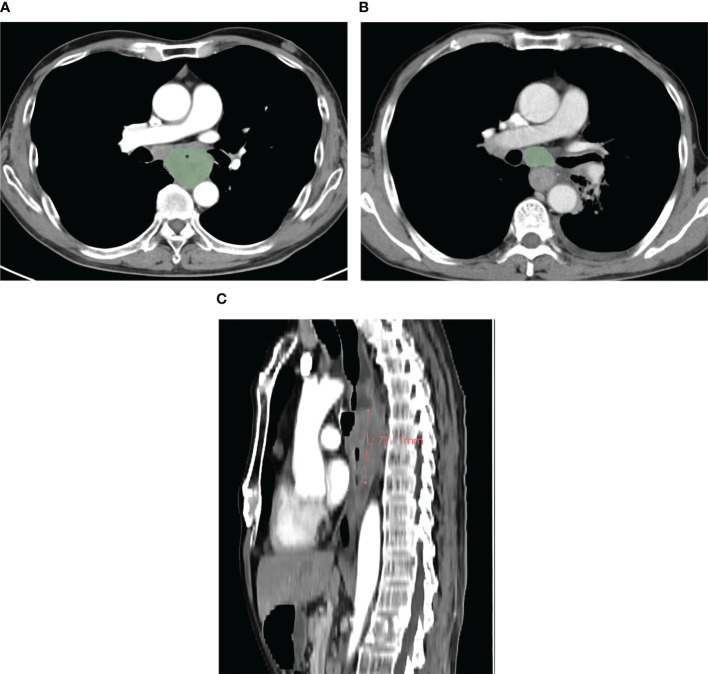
In a 59-year-old male patient with cT_4_N_1_M_0_ esophageal squamous cell carcinoma, the pretherapeutic contrast-enhanced CT scans show the gross tumor volume obtained by slice-by-slice manual delineation of the tumor (A), and the gross tumor volume is 57.66 cm^3^. In a 64-year-old male patient with esophageal squamous cell carcinoma at cT_3_N_1_M_0_, the gross volume of the enlarged lymph nodes was obtained in a way similar to that of the tumor (B), and the gross volume of all enlarged lymph nodes is 21.28 cm^3^. In a 59-year-old male patient with cT_4_N_2_M_0_ esophageal squamous cell carcinoma, the tumor length was obtained with the value of 7.11 cm (C).
